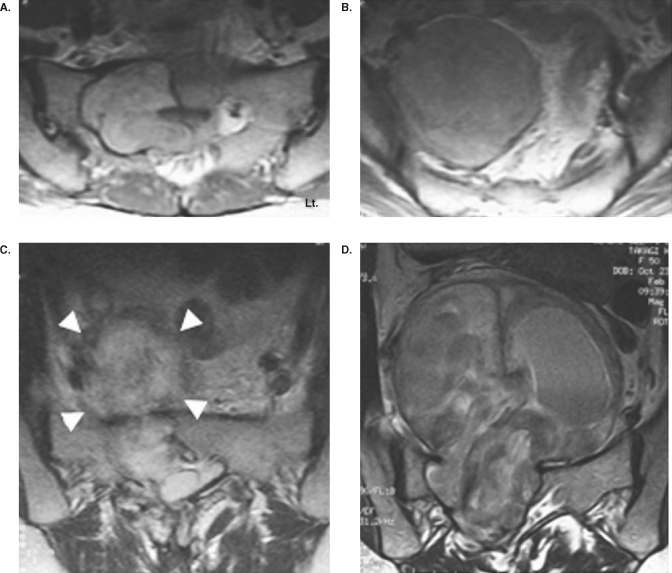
Axial image of T2-weighted MRI showing a big tumor extending from the spinal canal through the sacral body of S1 (A) to the presacral region (S2 level (B)) before surgery. The tumor was partially removed via a combination of the anterior and posterior approach. Axial image of T2-weighted MRI immediately after the surgery (C) showing a postoperative hematoma in the remaining tumor capsule (white arrow-head). Seven years after the surgery, the remaining tumor grew (D), and complete removal of the tumor with S1 root sacrifice was performed.
